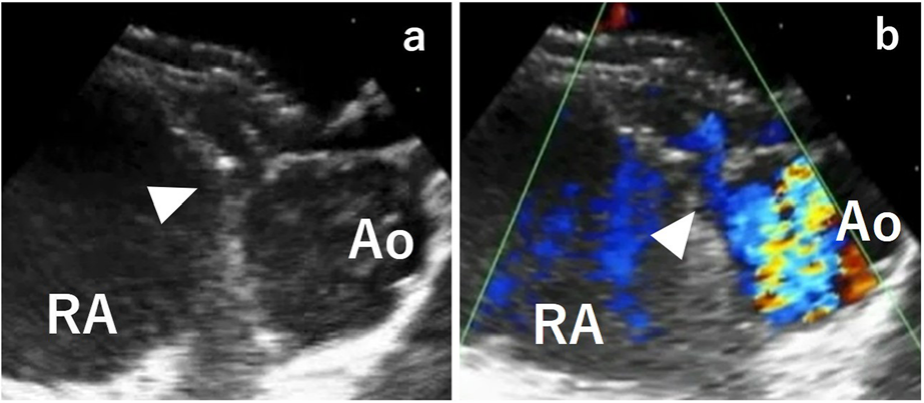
a, b. The transesophageal echocardiogram during the emergency operation for cardiac tamponade. The edge of the Amplazter septal occluder was touching the aortic wall (a. white arrowhead) and Doppler color-flow imaging showed blood flow through this lesion of the aorta (b. white arrowhead)
